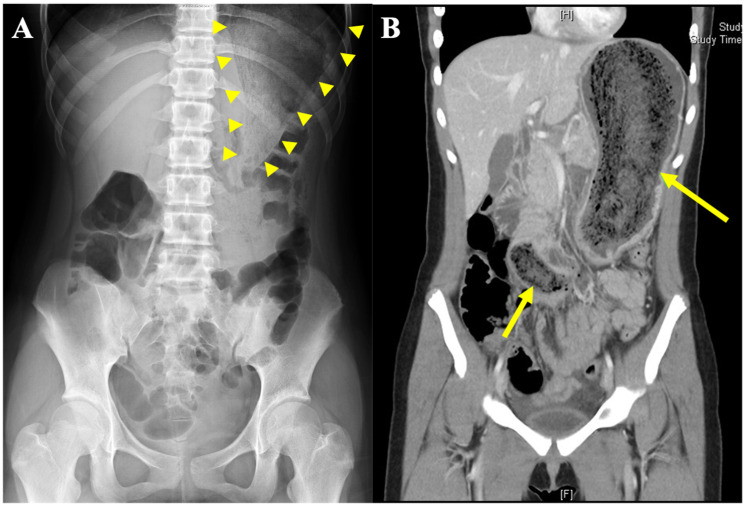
(A) Abdominal X-ray of a patient with a trichobezoar, with yellow arrowheads indicating the trichobezoar within the stomach. (B) Abdominal CT scan of the same patient, with yellow arrows pointing to the trichobezoar extending through the stomach and duodenum, interspersed with air and mottled calcifications (H: head end; F: foot end).
